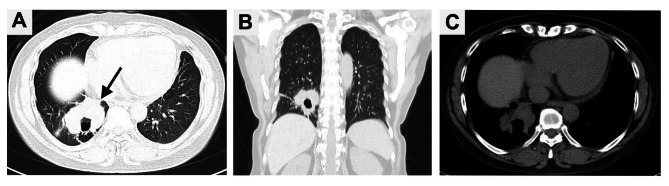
Follow-up CT examination on June 7, 2022 showed that the mass continued to enlarge and the solid part increased, and its size was about 58 mm×63 mm (black arrow). (A) Axial lung window; (B) Coronal lung window; (C) Axial soft tissue window
